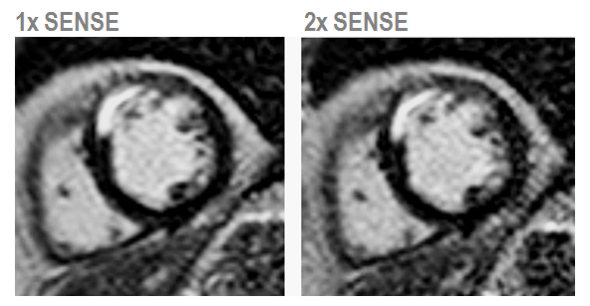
Comparison of 2D late-contrast enhanced MR imaging without and with 2× SENSE acceleration and using a 6-element coil array. Both images provide similar image quality with clear delineation of an antero-septal scar. The SNR decrease is seen with 2× SENSE, best appreciated in the lateral wall.
